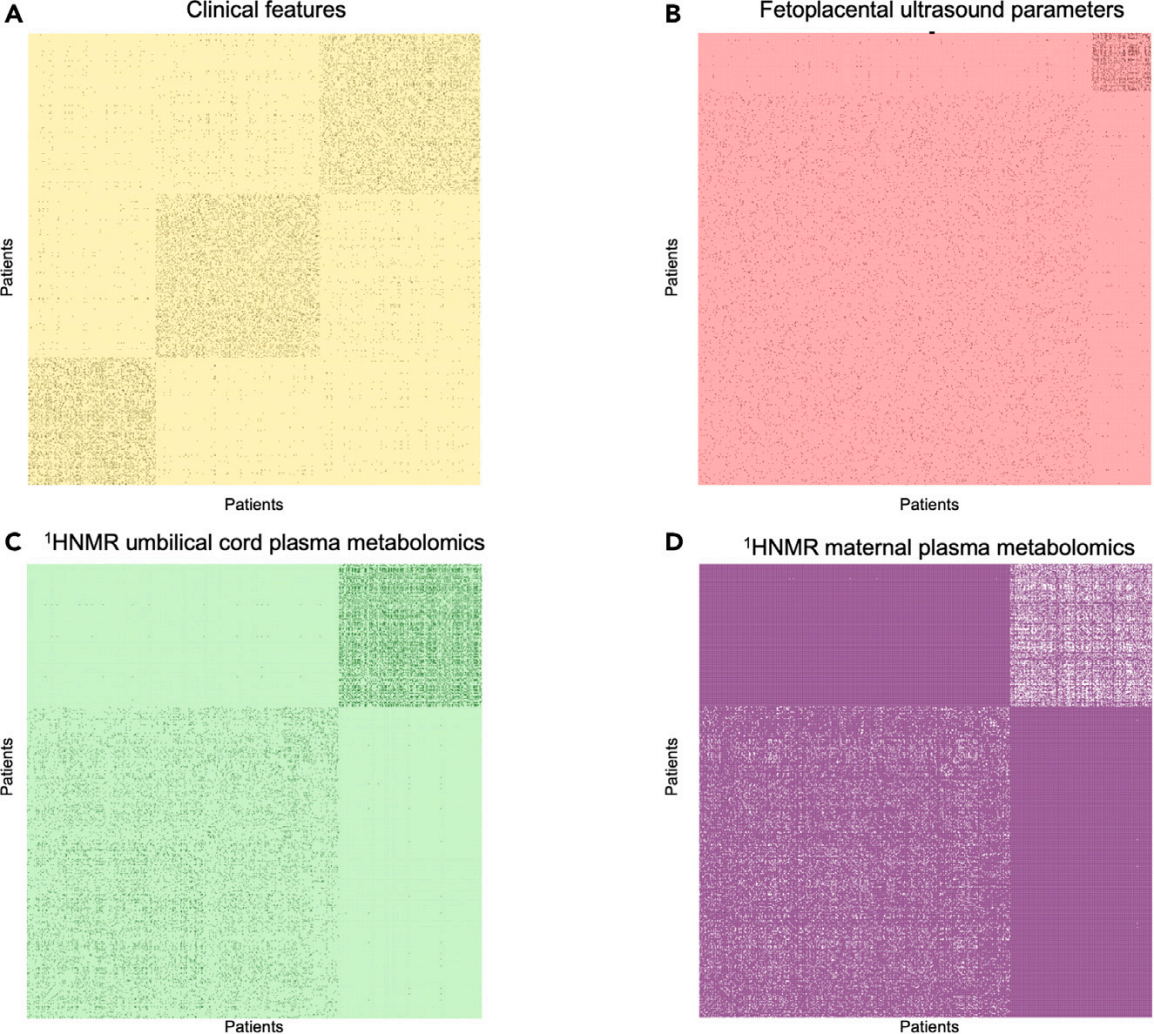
Distinct patient grouping within single omic networks (A) Heatmap of similarities between pairs of patients (both axes; same order from bottom-left) for maternal clinical features (blood pressure, angiogenic factors, and gestational age at diagnosis). The groups are visually depicted using spectral clustering that draws the groups of patients to the diagonal of the heatmap; the more substantial the similarity between patients, the closer they are grouped, and the lighter the color is shown. (B) Similarity network using maternal-fetal ultrasound (fetal biometry and maternal-fetal Doppler). (C and D) Similarity network using cord blood metabolomics, and (D). Similarity network using metabolomic fingerprinting in maternal blood.
